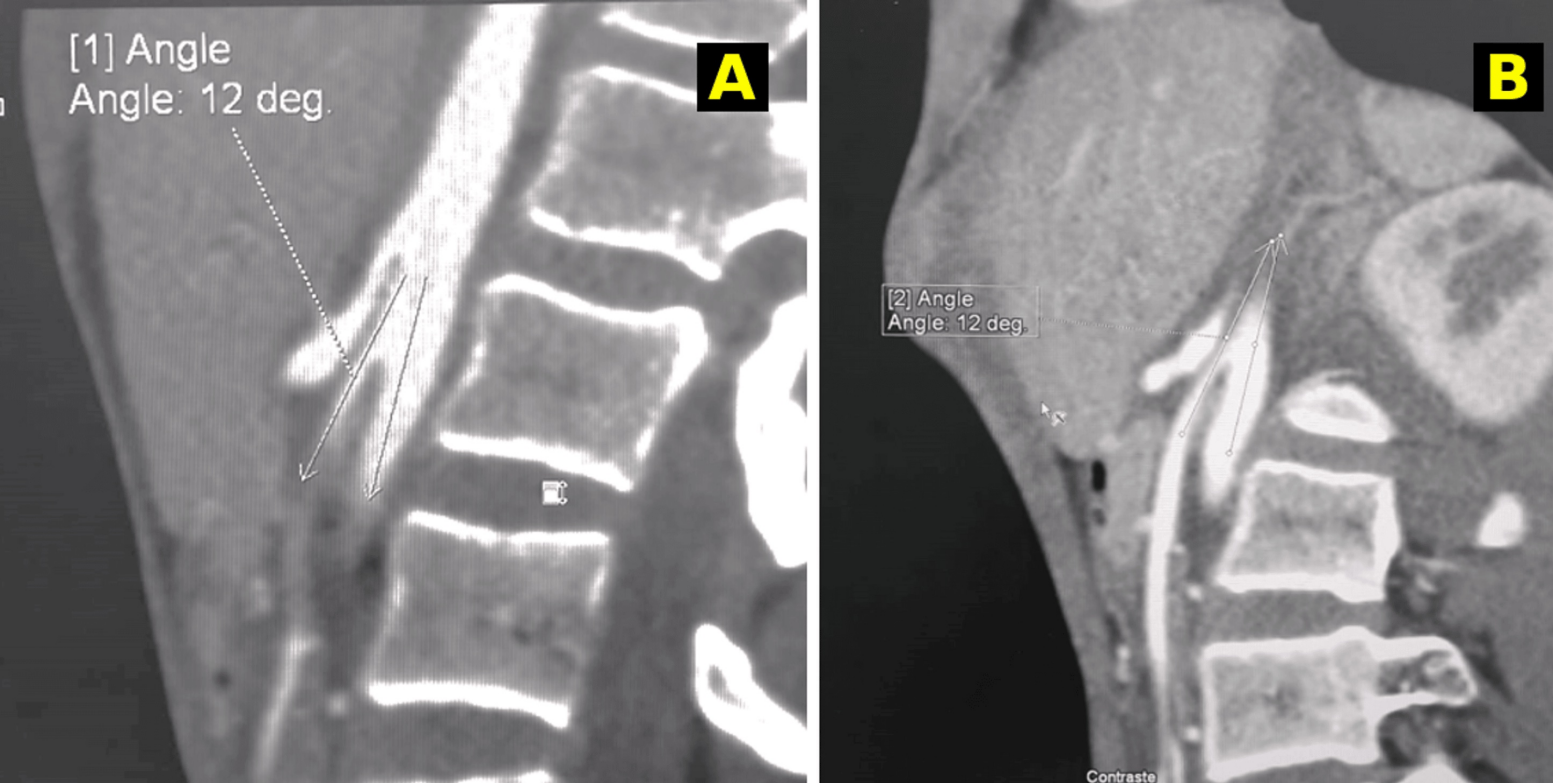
Sagittal section of an abdominal-pelvic CT scan after contrast injection. Sagittal reconstruction from a contrast-enhanced abdominal CT scan demonstrating a markedly reduced aorto-mesenteric angle, measured at 12°, consistent with superior mesenteric artery (SMA) syndrome. (A) Sagittal CT slice showing the compression of the third portion of the duodenum between the abdominal aorta and the SMA, with measurement lines indicating the narrowed angle. (B) Another sagittal view confirming the aorto-mesenteric angle reduction to 12°, supporting the diagnosis of SMA syndrome.
